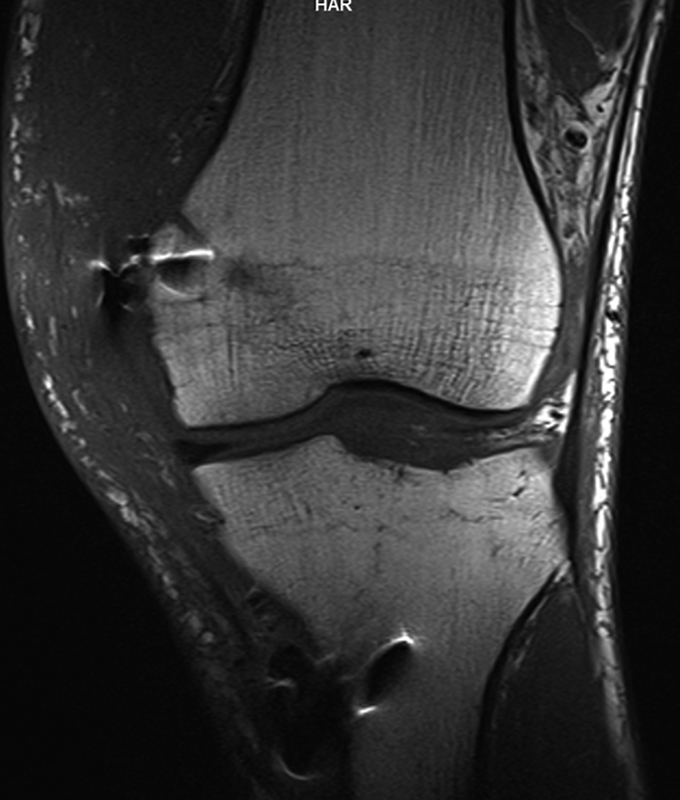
Magnetic resonance imaging showing the resolution of the bone edema.
